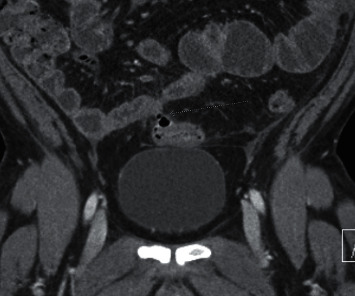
Coronal CT enterography image of the lower abdomen demonstrates a sinus tract adjacent to the extraluminal collection which bridges an air-filled sigmoid diverticulum and the adjacent distal ileal loop.
